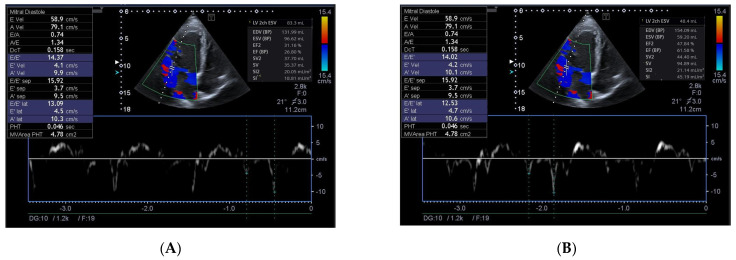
An example of the determination of HFimpEF in patients with a 3-month interval between investigations. (A) Echocardiographic parameters at baseline. (B) Echocardiographic parameters in 3 months.
